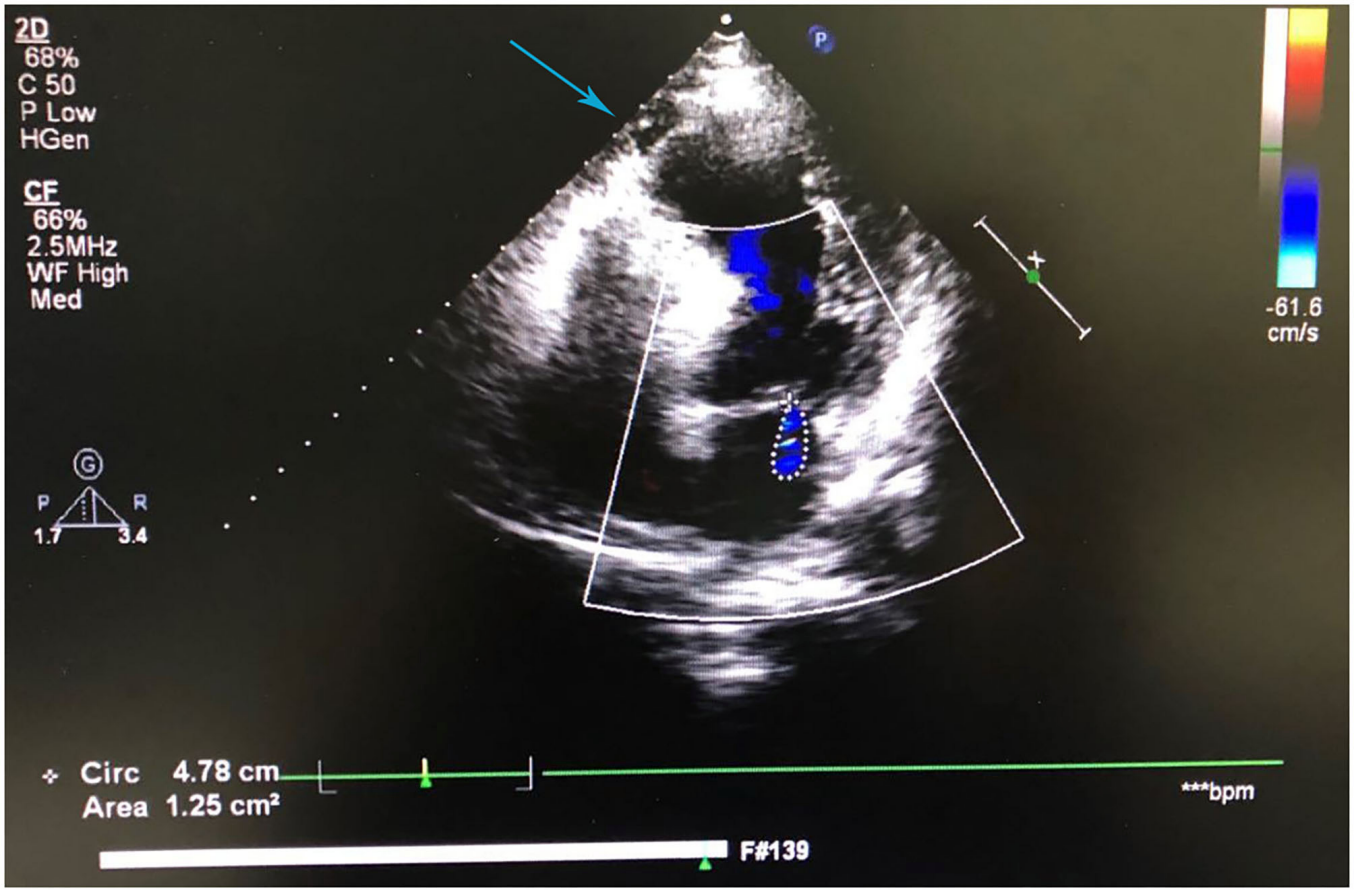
Color Doppler echocardiography shows left ventricular apical aneurysm formation and left ventricular systolic and diastolic dysfunction.
